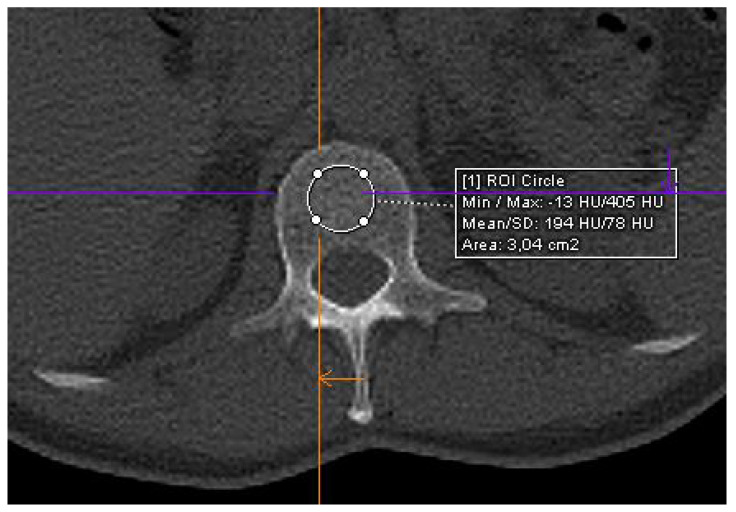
Axial CT image showing ROI placement at the level of the trabecular bone of the L1 vertebra. Purple and orange lines are for anatomical reference of correct positioning.
